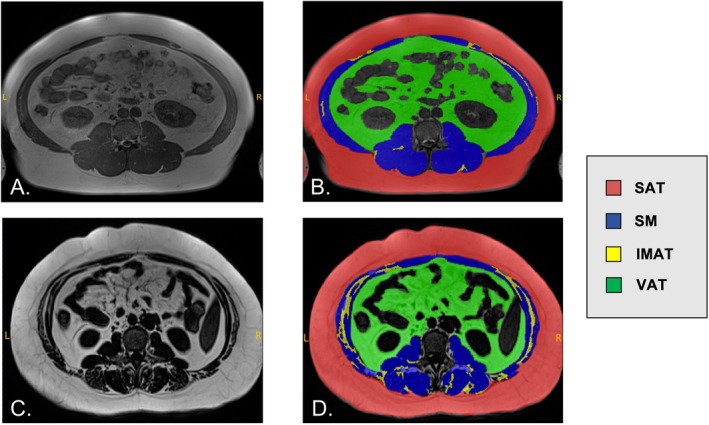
MRI‐based IMAT imaging in patients with comorbid type 2 diabetes mellitus and non‐alcoholic fatty liver disease. (A) Original axial MRI image of a 35‐year‐old male with type 2 diabetes mellitus and non‐alcoholic fatty liver disease, obtained at the abdominal level for body composition evaluation. (B) Corresponding segmented image with colour‐coded SAT (red), SM (blue), IMAT (yellow) and VAT (green). (C) Original axial MRI image of a 40‐year‐old female with type 2 diabetes mellitus and non‐alcoholic fatty liver disease, acquired at a comparable anatomical level for body composition assessment. (D) Corresponding segmented image with colour‐coded SAT, SM, IMAT and VAT. Both cases demonstrate prominent IMAT deposition interleaved within skeletal muscle bundles alongside expanded visceral adipose tissue, reflecting the adverse ectopic fat phenotype characteristic of concurrent metabolic dysfunction. Cross‐sectional areas were segmented using dedicated body composition software. IMAT = intermuscular adipose tissue; MRI = magnetic resonance imaging; SAT = subcutaneous adipose tissue; SM = skeletal muscle; VAT = visceral adipose tissue.
